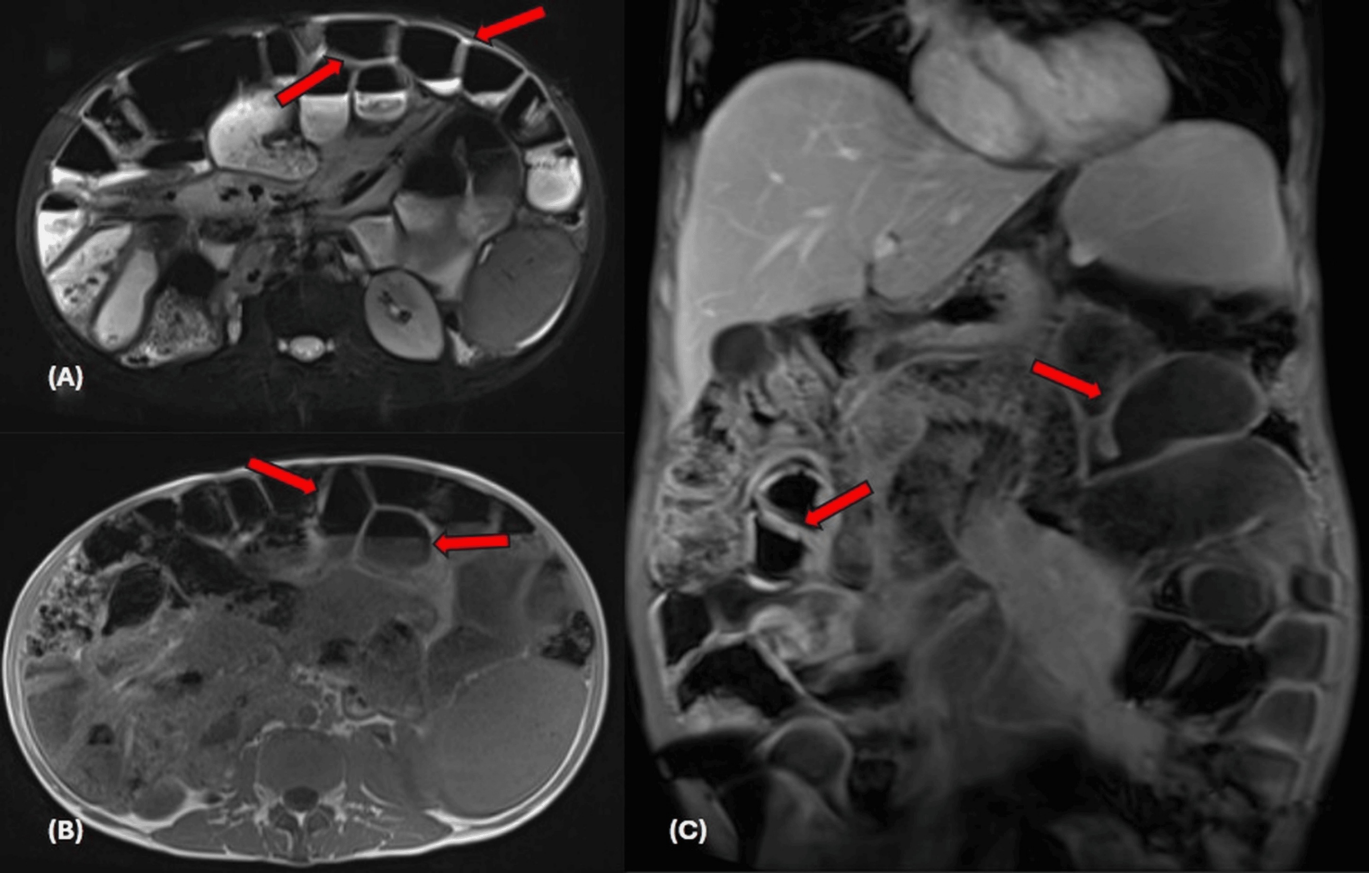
MRI abdomen with contrast Axial T2 image (A), axial post-contrast T1, and coronal T1 post-contrast, showing diffuse circumferential small bowel thickening.
